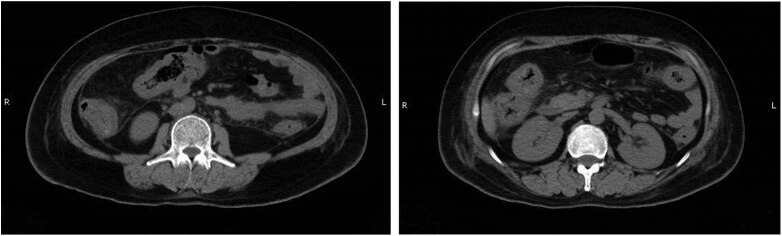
CT scan images on September 7, 2022. After CHM treatment, the cecum and descending colon were normal, while there was a small effusion of blood in the abdominal cavity. There is an edematous thickening of the ascending and transverse colon.
